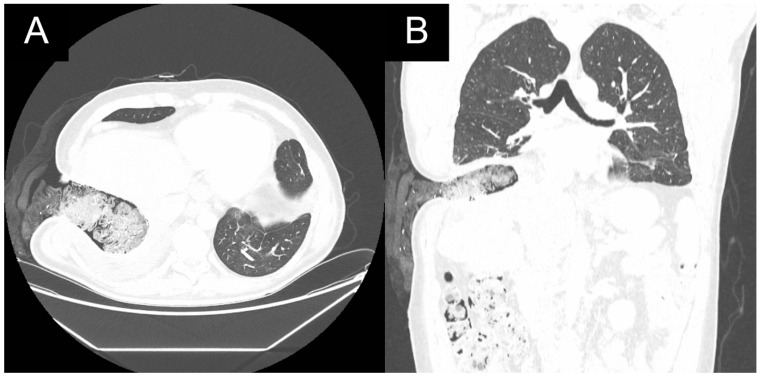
Postoperative chest CT in (A): axial and (B): coronal views of patient 1 with open window thoracostomy.
